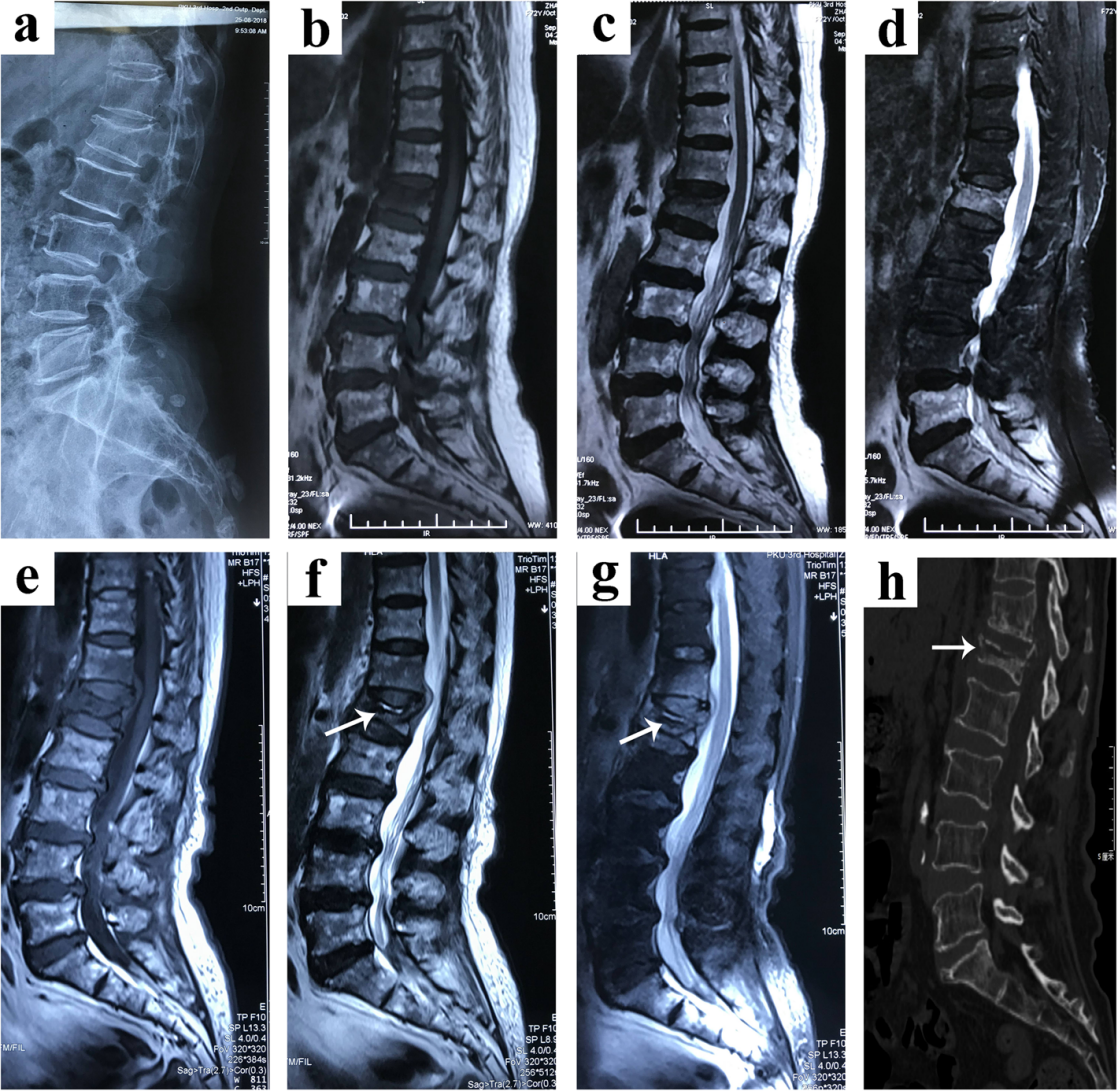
Preoperative X-ray, CT and MRI of the patient. a lateral radiographs show a vertebral collapse of L1 three months before surgery. b-d Sagittal MR images of lumbar spine display L1vertebral collapse without IVC three months before surgery. e-f Preoperative sagittal MR images show a fluid-containing IVC with high-signal intensity on T2-weighted images and STIR MR sequences. h Preoperative sagittal reconstruction CT scan shows a linear radiolucent shadow that is located adjacent to the upper endplate of L1collapsed vertebral body
